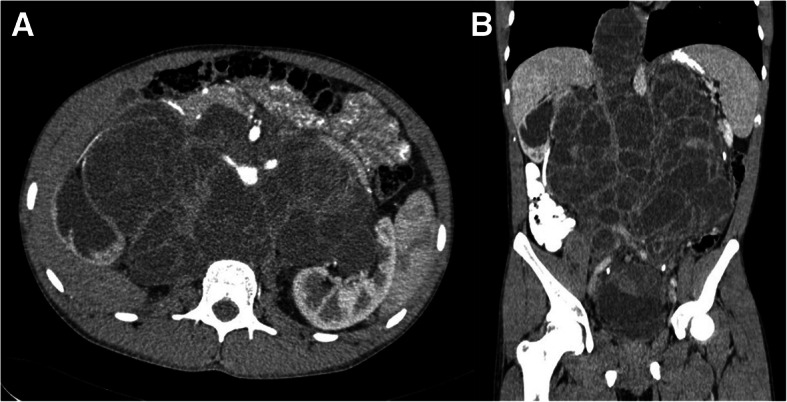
CT scans confirming giant retroperitoneal tumor extending to the posterior mediastinum. a – axial, b – coronal
